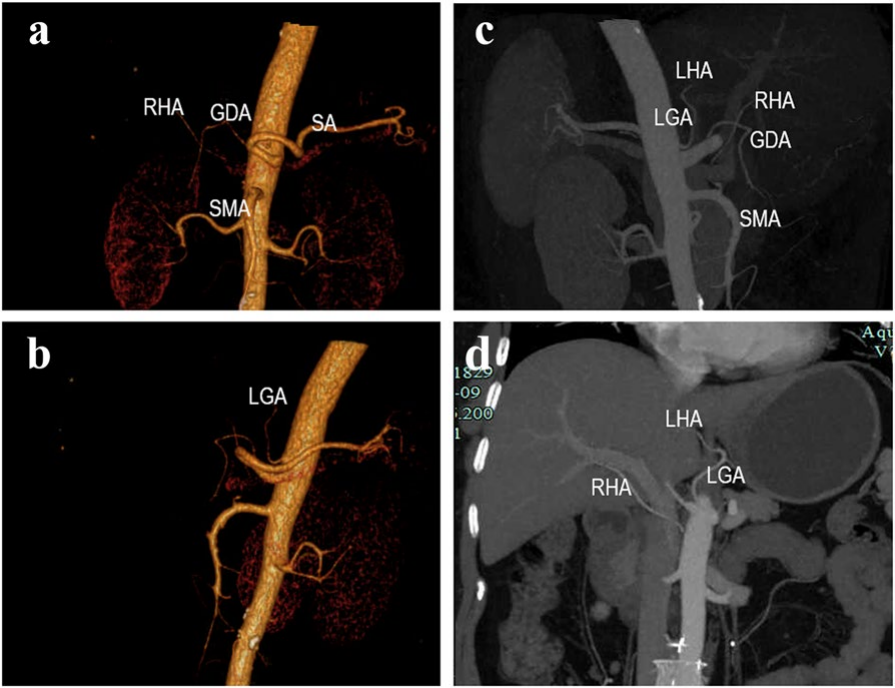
Computed tomography angiography (c, d) with reconstruction (a, b). An absence of the celiac trunk artery (CA) and the common hepatic (CHA) artery. The splenic (SA) and gastroduodenal (GDA) originates from the AA. The left gastric (LGA) originates from the abdominal aorta directly above the junction of the SA and GDA. At the same time, the left hepatic artery (LHA) originates from the LGA. The right hepatic artery (RHA) originates from the superior mesenteric artery
